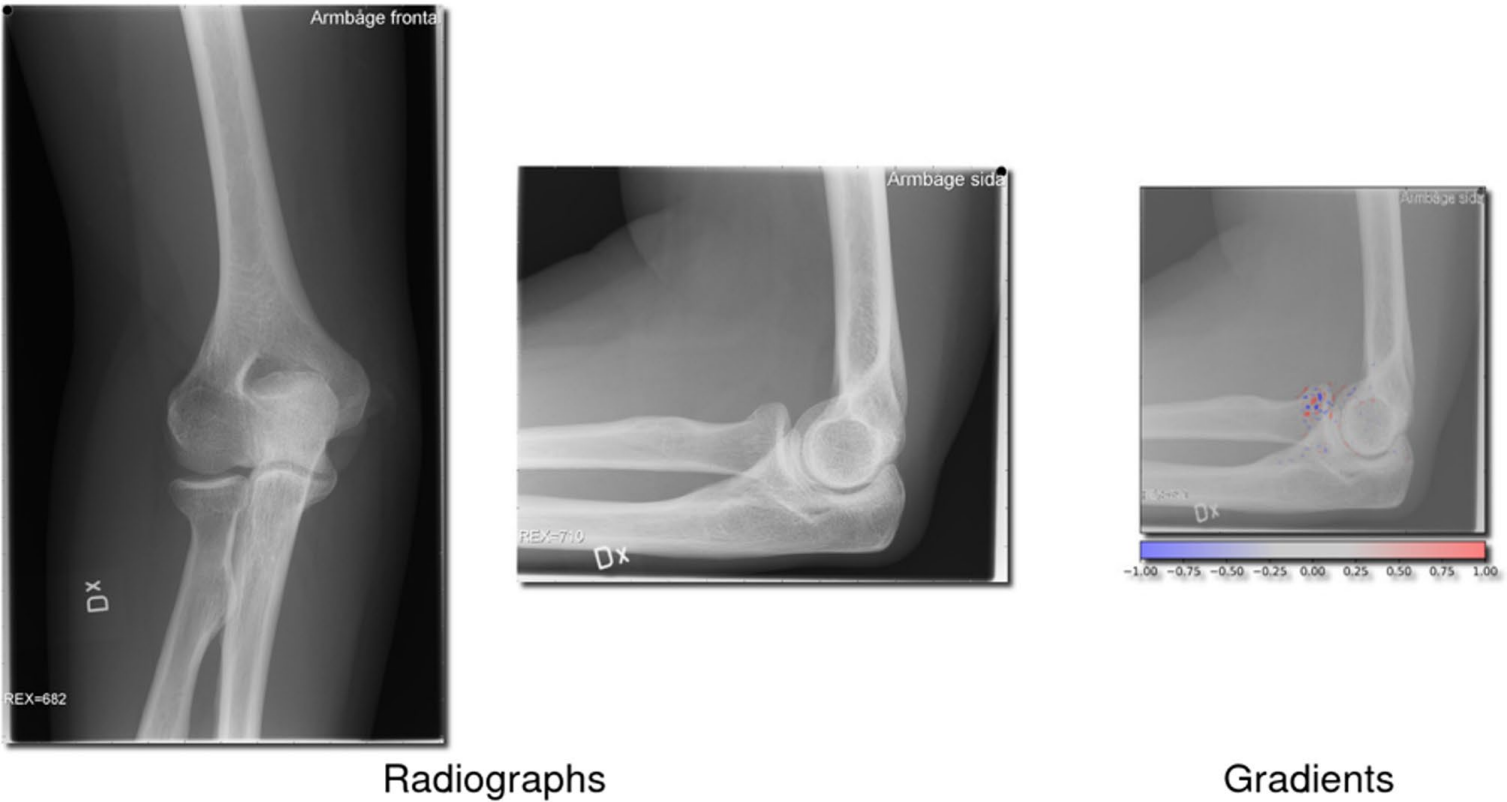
Missed proximal radius (2R1A) fractures. This figure showcases 2 radiographs in which the network failed to detect a 2R1A fracture, and 1 radiograph with gradients being overlayed showing the network’s interpretation
